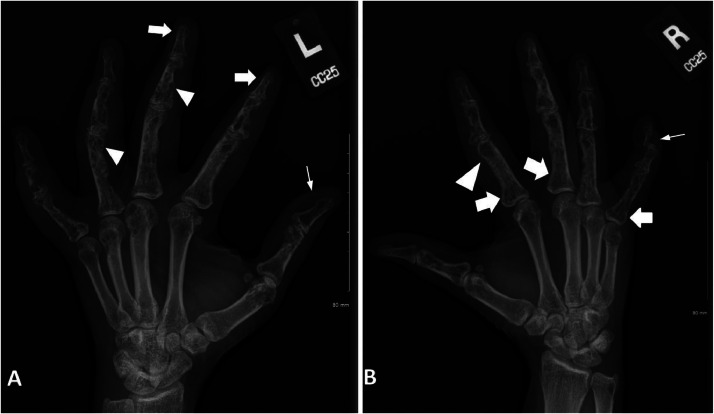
(A) Oblique radiograph of the left hand demonstrates multiple, well-delineated, small round lucencies throughout the phalanges (white arrowhead) with bony erosions predominantly in the first distal phalanx (white arrow) and second through fourth middle phalanges. “Lace-like” reticulation is noted at the tufts of the 2nd and 3rd phalanges (wide white arrows). Diffuse soft tissue swelling is noted along the digits. (B) Oblique radiograph of the right hand demonstrates similar findings of multifocal round lucencies with bony erosions predominantly affecting the fifth distal phalanx (white arrow). Note the solitary focal lucency in the distal ulna. Larger, bullous cyst-like lesion in the second distal phalanx (white arrowhead). Similar “lace-like” reticulation is noted at the base of multiple proximal phalanges (wide white arrows).
